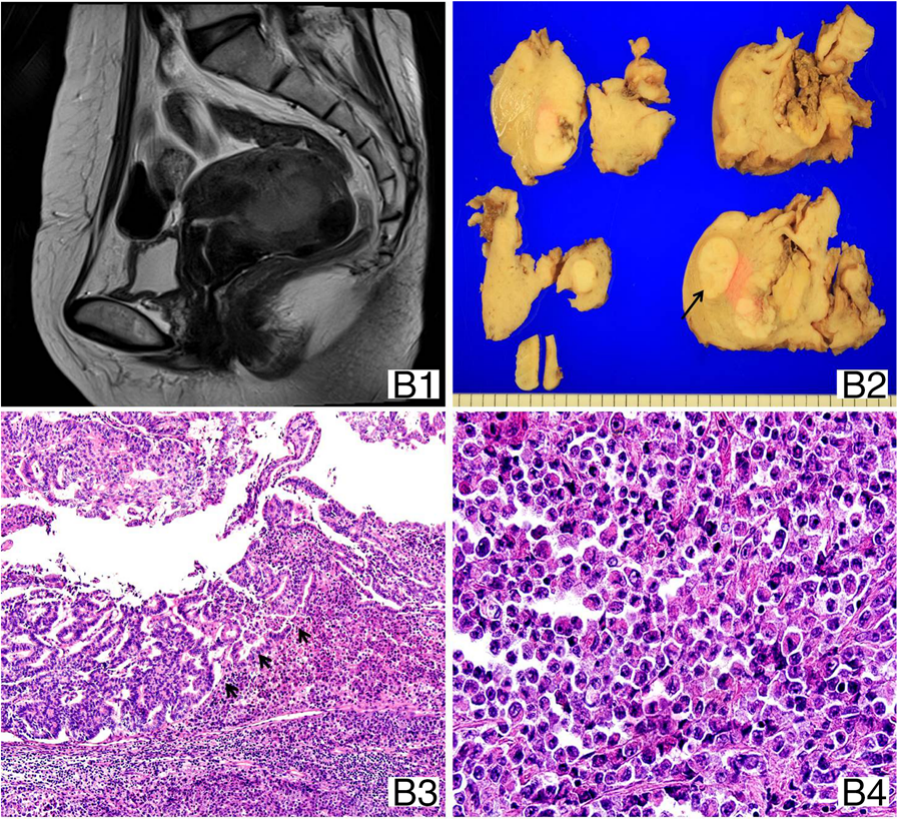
Case 2. (*B1*) Sagittal T2-weighted MRI shows a large polypoid endometrial mass with superficial infiltration of myometrium. (*B2*) Gross photograph shows a polypoid mass compacting the endometrial cavity. Well-circumscribed round mass, submural leiomyoma is seen (*arrow*). (*B3*, ×100) The tumor comprises moderately differentiated endometrioid adenocarcinoma and UC with abrupt transition (*arrows*) between them. (*B4*, ×400) The UC cell component shows discohesive rhabdoid feature
